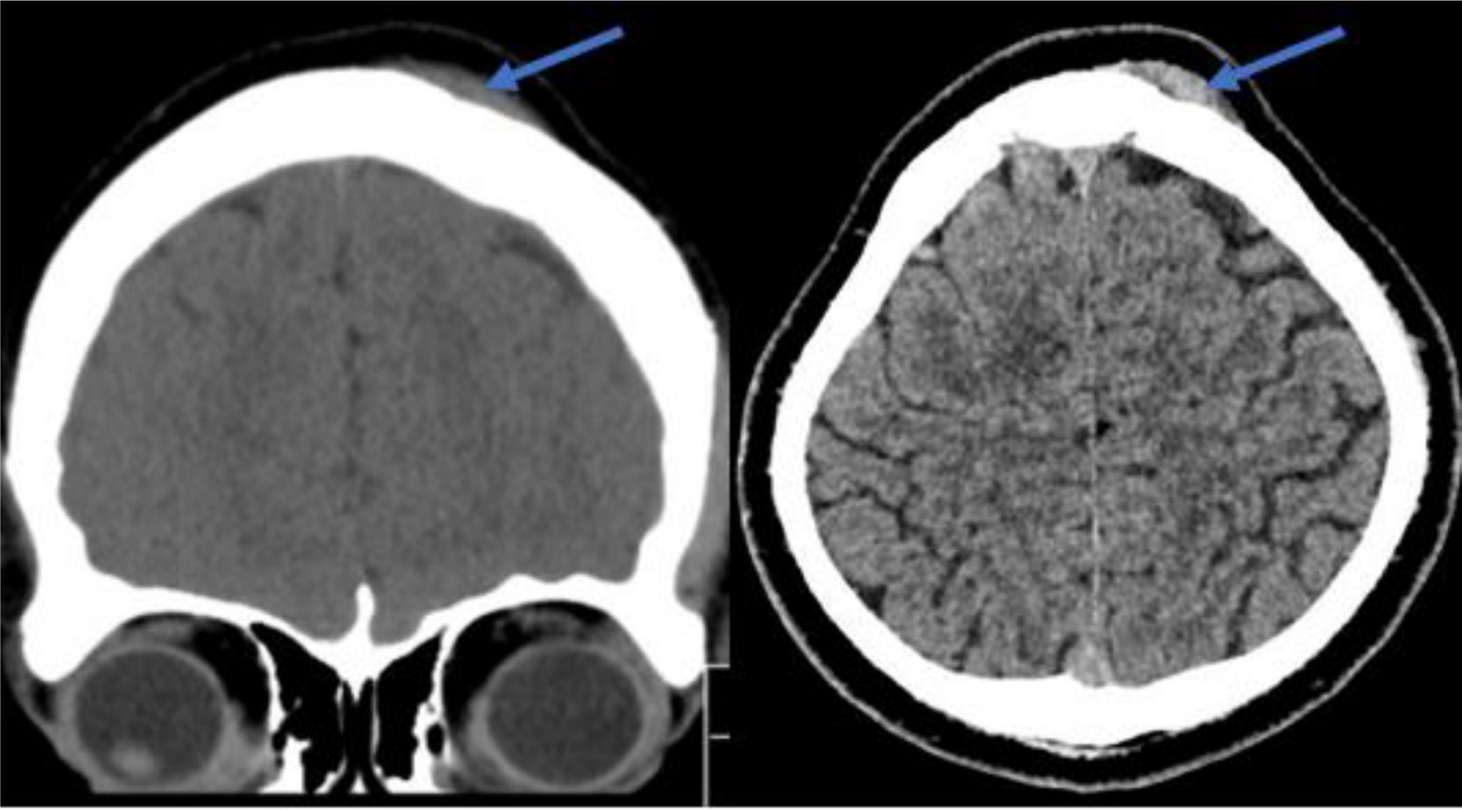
CT scan showing subcutaneous isodense lesion at the left frontal part of the scalp (blow arrows) causing erosion of the underlying bone.
